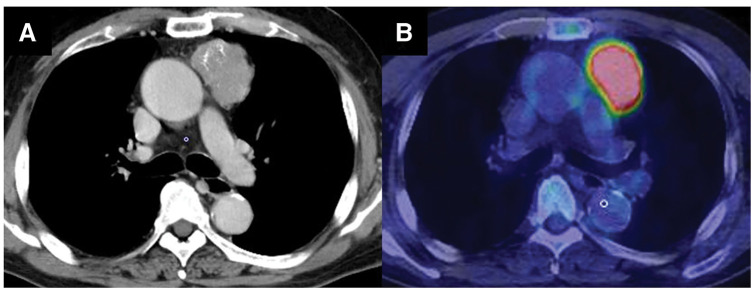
Preoperative images of the tumor. (A) Chest CT reveals a 5.1 × 5.0-cm well-defined mass in the left anterior mediastinum with calcification. (B) Fluorodeoxyglucose-PET shows increased fluorodeoxyglucose uptake with a maximum standardized uptake value of 12.0.
